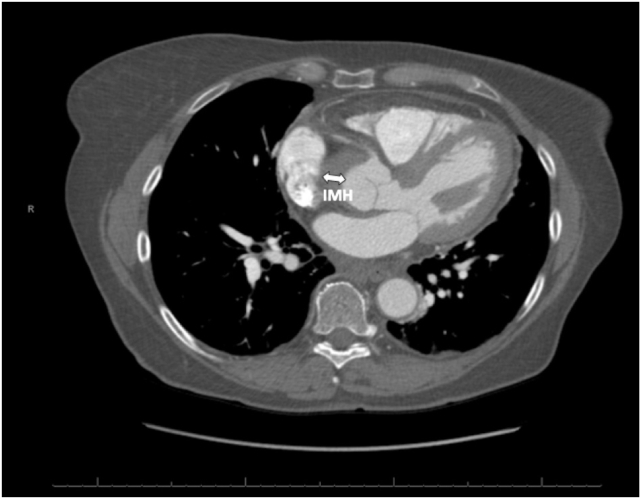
Contrast-enhanced CT scan, axial display at the sinus of Valsalva, demonstrates the proximal location of the IMH (*arrow*) adjacent to the origin of the right coronary artery.
